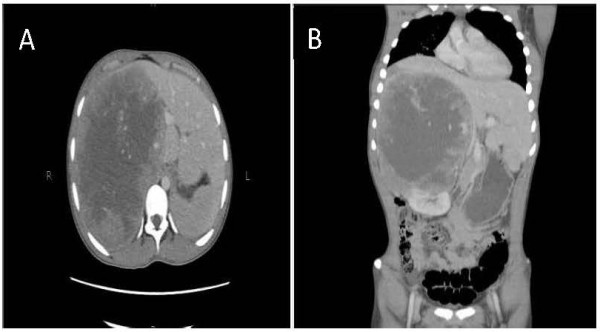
Computed tomography scan of the abdomen at presentation. Large mass arising from the right adrenal gland visible in axial section (A) and coronal section (B).
